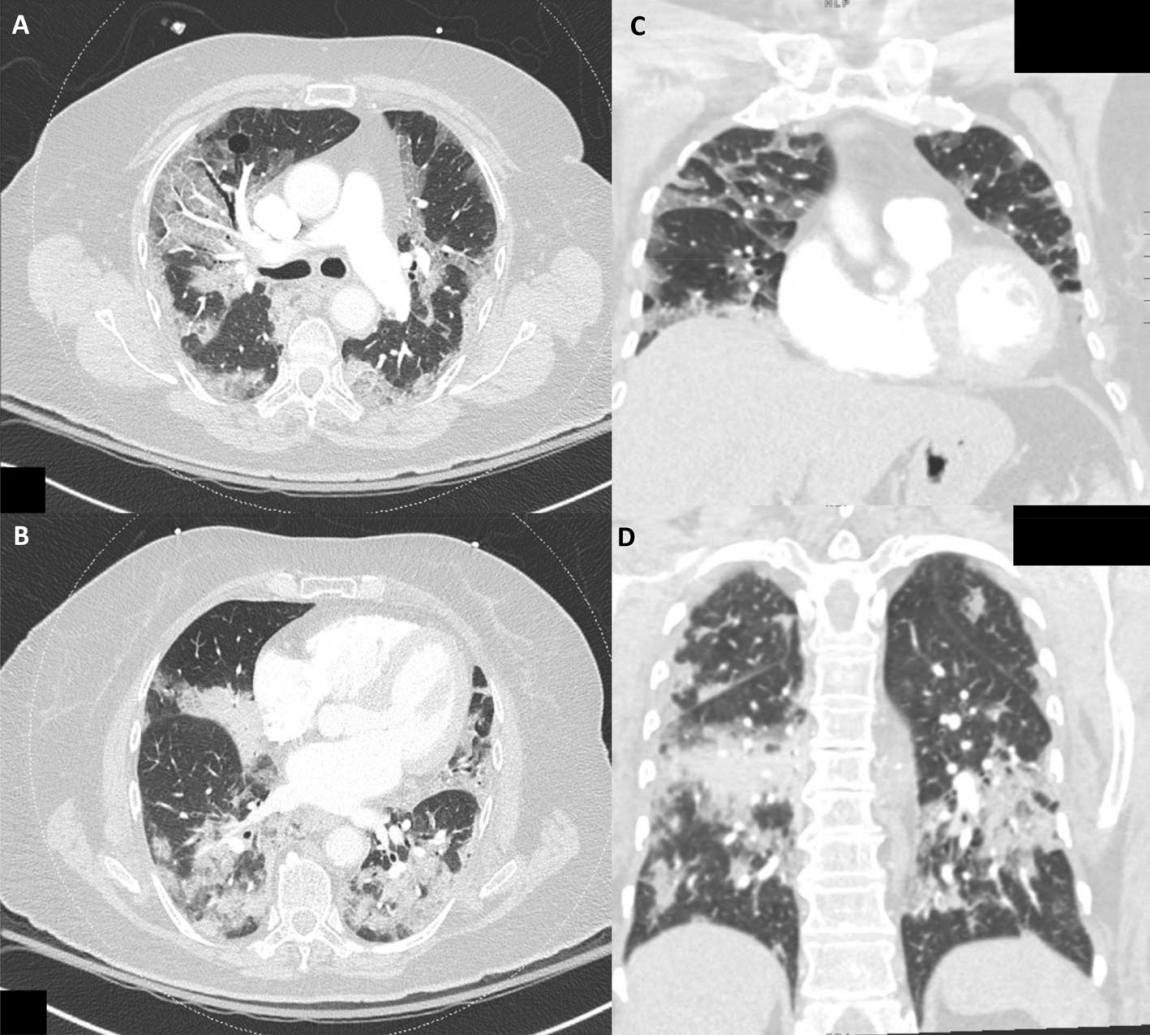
Computed tomography images of the chest from the COVID‐19 patient who received lung ultrasonography to track lung surface movement on the same day. (A) Axial image of the upper lungs at the approximate level corresponding to upper lung ultrasound zones. (B) Axial image of the lower lungs corresponding to the lower lung ultrasound zones. (C) Coronal image of the anterior lungs. (D) Coronal image of the posterior lungs.
